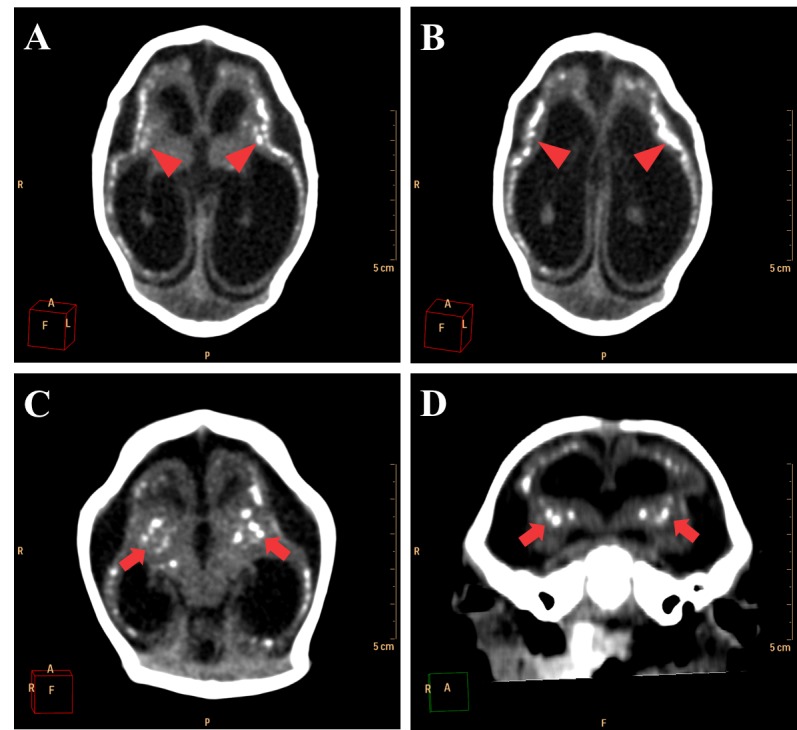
Figure 2. Most frequent radiological findings on CT. Axial (A, B and C) and coronal (D) non-contrast brain CT. A and B show a 1 month old infant female with evidence of severe microcephaly with subcortical gross calcifications (red arrowheads), accentuated compensatory lateral ventricles dilation and loss of typical sulcation configuring lisencephaly. (C and D) Gross calcifications in the basal ganglia (red arrows) in a 2 months old female newborn, configuring one of the most typical findings of this presentation.
